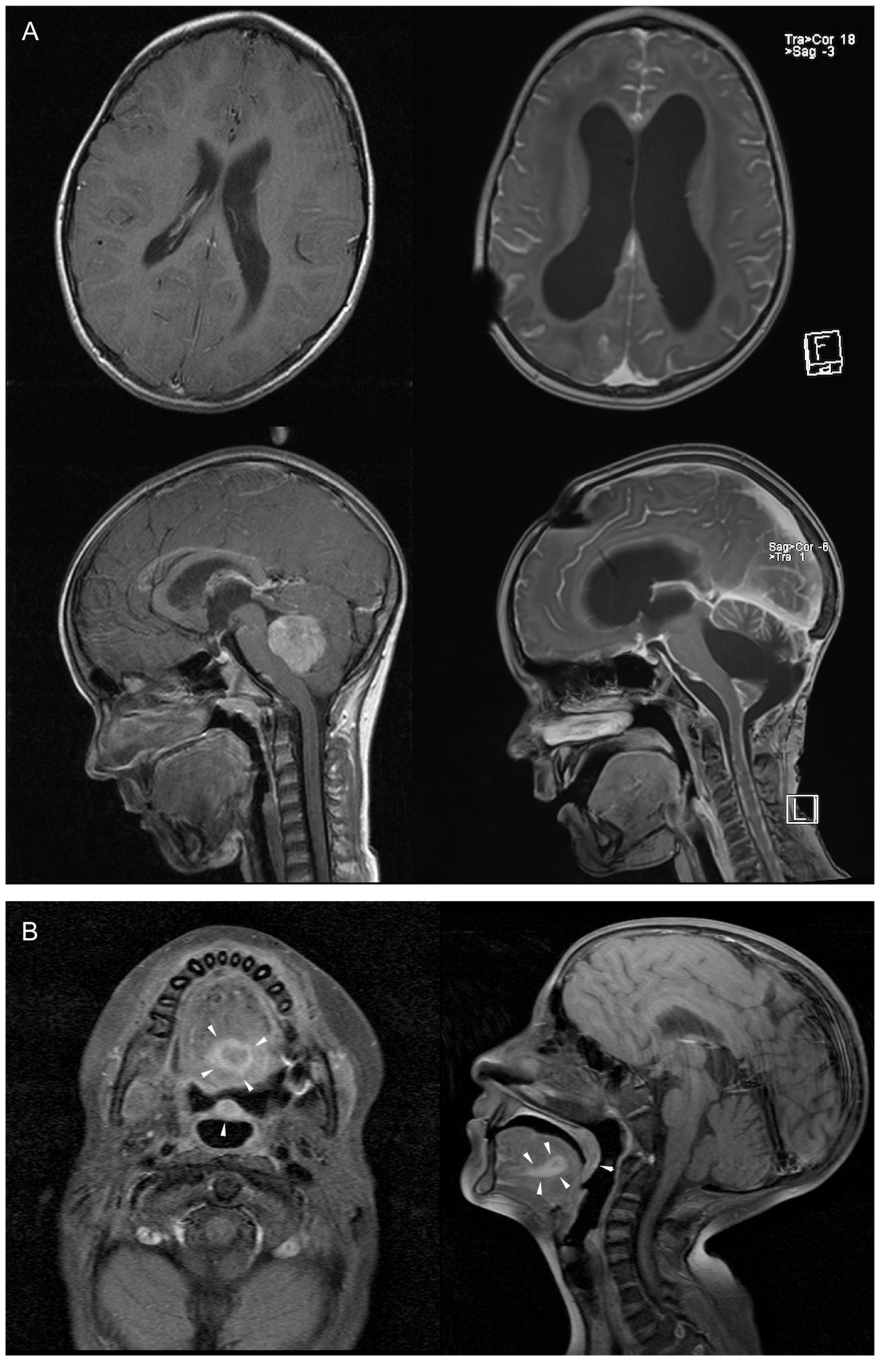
MR imaging documenting severe normal tissue toxicity or synchronous tumors in patients. (A) Cranial magnetic resonance imaging of the patient GRJN before (left panel) and after tumour resection and adjuvant radio-/chemotherapy (right panel). T1-weighted images acquired in sagittal and axial plane shows cerebral atrophy with enlargement of the ventricles after multifactorial leukencephalopathy. (B) Cranial magnetic resonance imaging of the patient SBNE before surgical tumor resection. T1-weighted images acquired in sagittal and axial plane reveal the squamous cell carcinoma (SCC) of the oral cavity (tumors marked by arrows).
